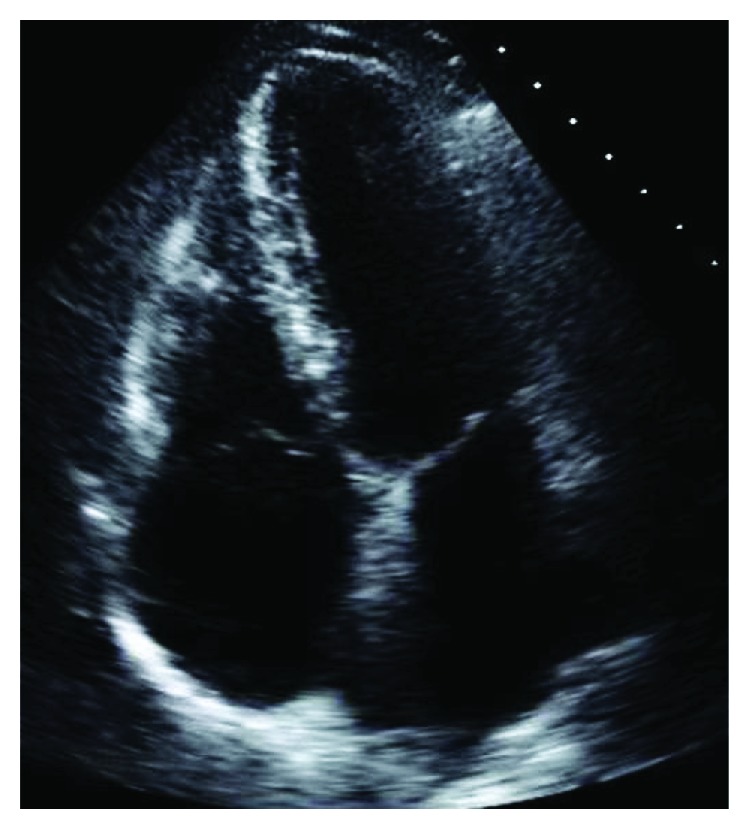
Apical four-chamber view of the heart with no evidence of a source of thrombosis.
